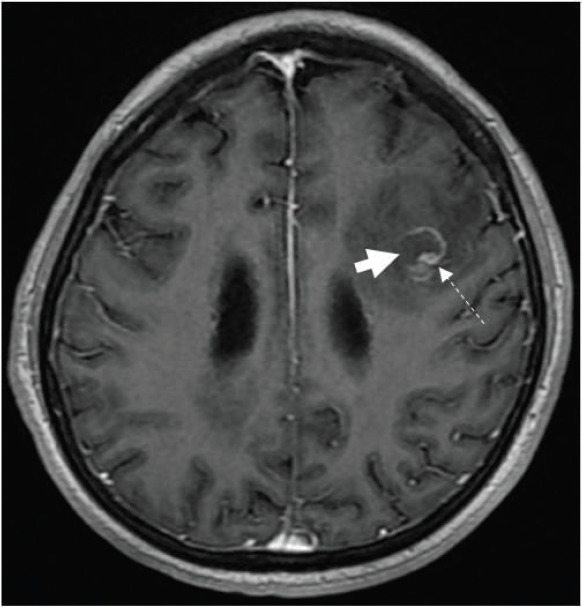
Axial T1-weighted post contrast-enhanced image showing an incomplete ring-enhancing lesion (short arrow) in the left frontal lobe. An eccentric focus of mural enhancement is seen (dashed arrow).
